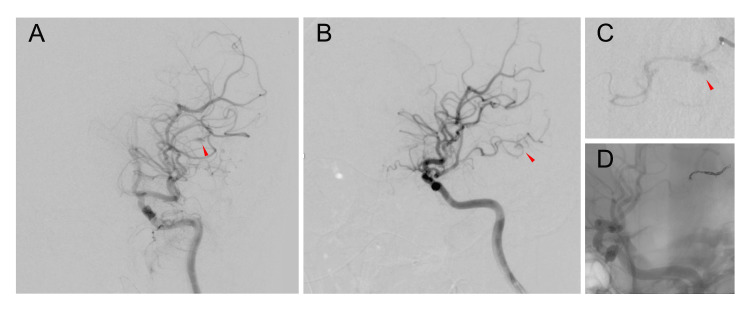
Pseudo-aneurysm detected in the digital subtraction angiography The anteroposterior (A) and lateral (B) views of the digital subtraction angiography of the left internal carotid artery revealed the aneurysm (arrowheads). The irregularly shaped aneurysm observed on computed tomography angiography was a pseudo-aneurysm (C, arrowhead), and the parent artery occlusion was performed (D).
